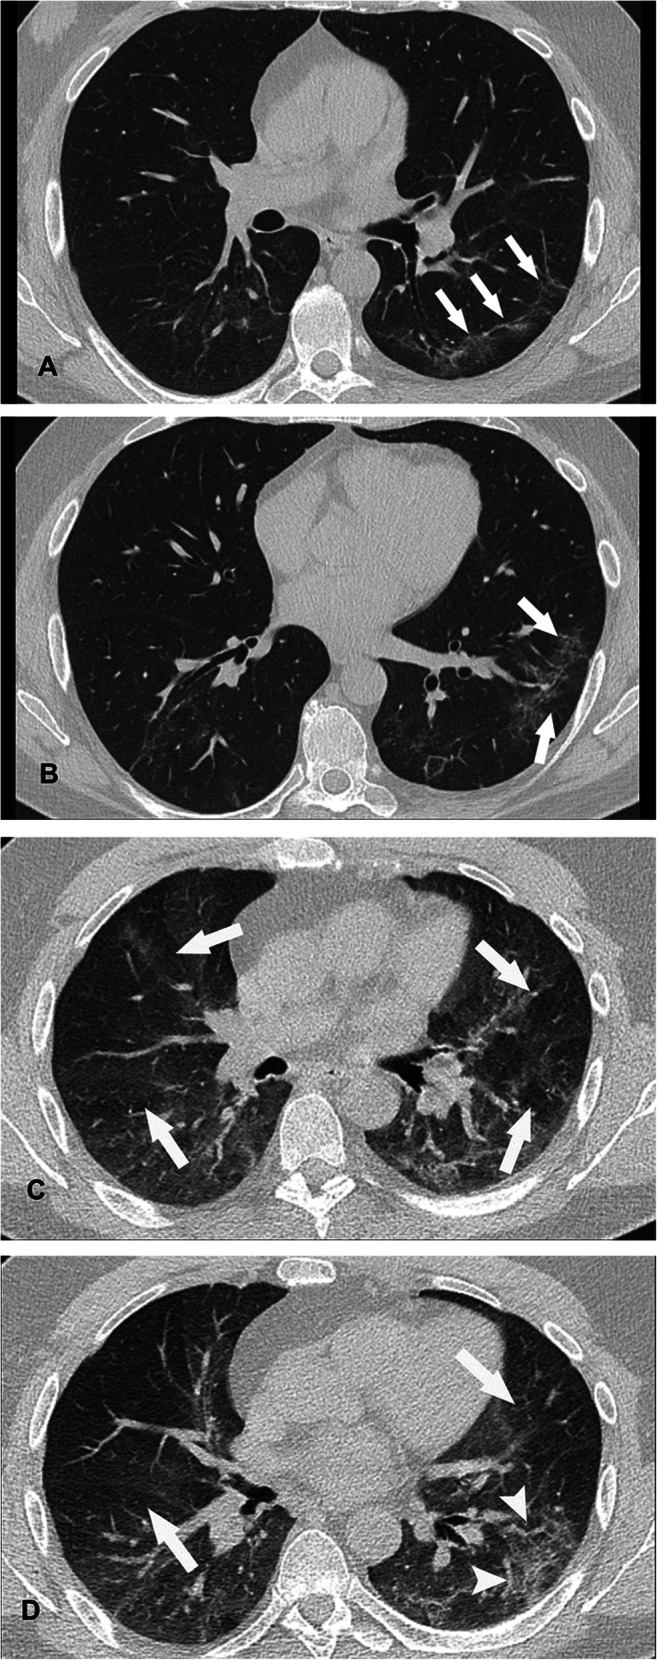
A, B Transverse CT scans in a 47-year-old nonsmoking man who had COVID-19 obtained during inspiration at follow-up examination, 70 days after dyspnea and shortness of breath, show minimal peripheral ground-glass opacities (arrows); C, D expiratory transverse CT images at the same levels show linear consolidation in the subpleural region of the left lower lobe (arrowheads) and bilateral multifocal areas of low attenuation due to air trapping (arrows) that takes up the entire segments. Note that the same levels appear normal on the inspiratory images
